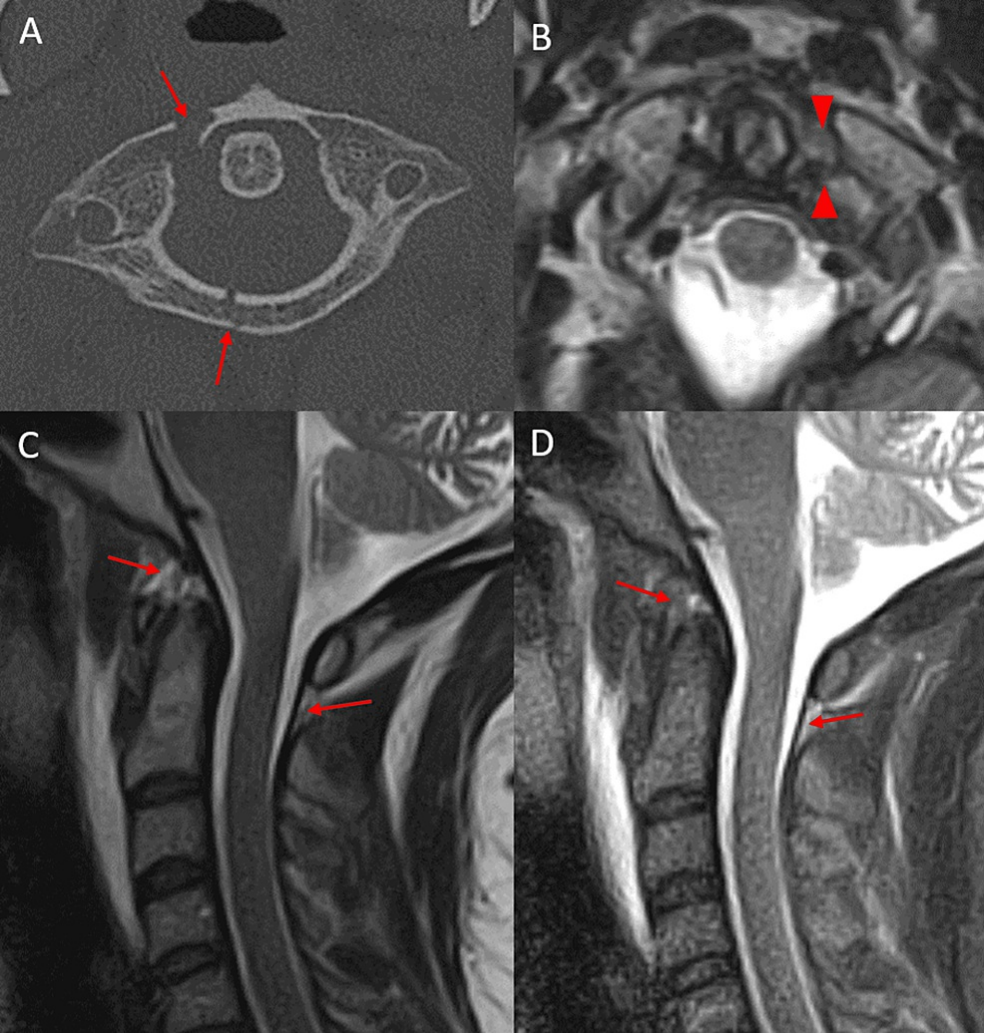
Axial CT of the cervical spine (A) in a 30-year-old trauma patient status post MVA demonstrating C1 anterior and posterior arch fractures (red arrows) and right lateral mass fracture. Axial T2-weighted MRI (B) in the same patient demonstrating a left transverse atlantal ligament tear (red arrowheads). Sagittal T2-weighted (C) and STIR-weighted (D) MRI of the cervical spine in the same patient demonstrating low-grade anterior and posterior atlanto-occipital membrane complex injuries (red arrows) with otherwise intact major craniocervical junction ligaments. Anterior and posterior atlanto-occipital membrane complex tears were relatively common in the setting of transverse atlantal ligament tears. CT: computed tomography; MVA: motor vehicle accident; MRI: magnetic resonance imaging; STIR: short tau inversion recovery
